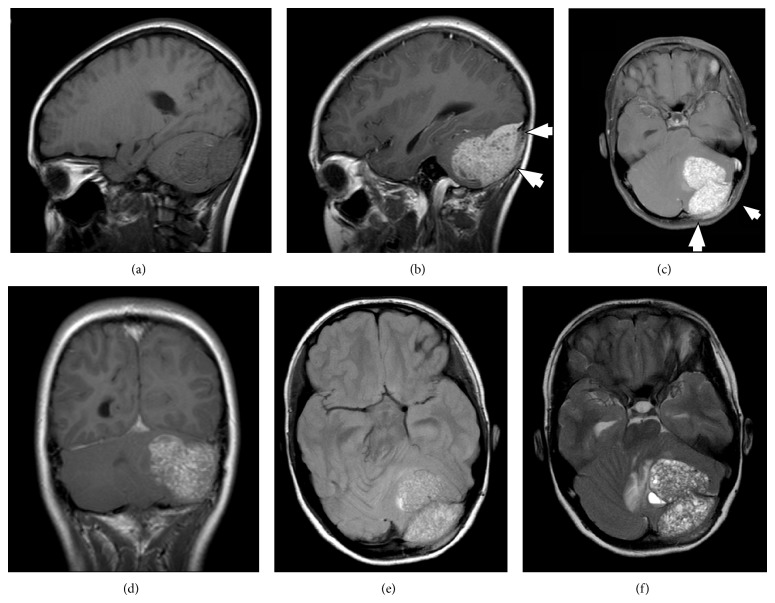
Preoperative MRI demonstrates a large contrast-enhancing dural-based tumor extending into the left posterior fossa forming two bulks. (a) T1-weighted noncontrast sagittal MRI. (b) T1-weighted contrast-enhanced sagittal view: short arrows indicate bone erosion. (c) T1-weighted contrast-enhanced axial MRI: short arrows indicate bone erosion. (d) T1-weighted contrast-enhanced coronary MRI. (e) Fluid attenuated inversion recovery- (FLAIR-) weighted coronal MRI. (f) T2-weighted axial view.
